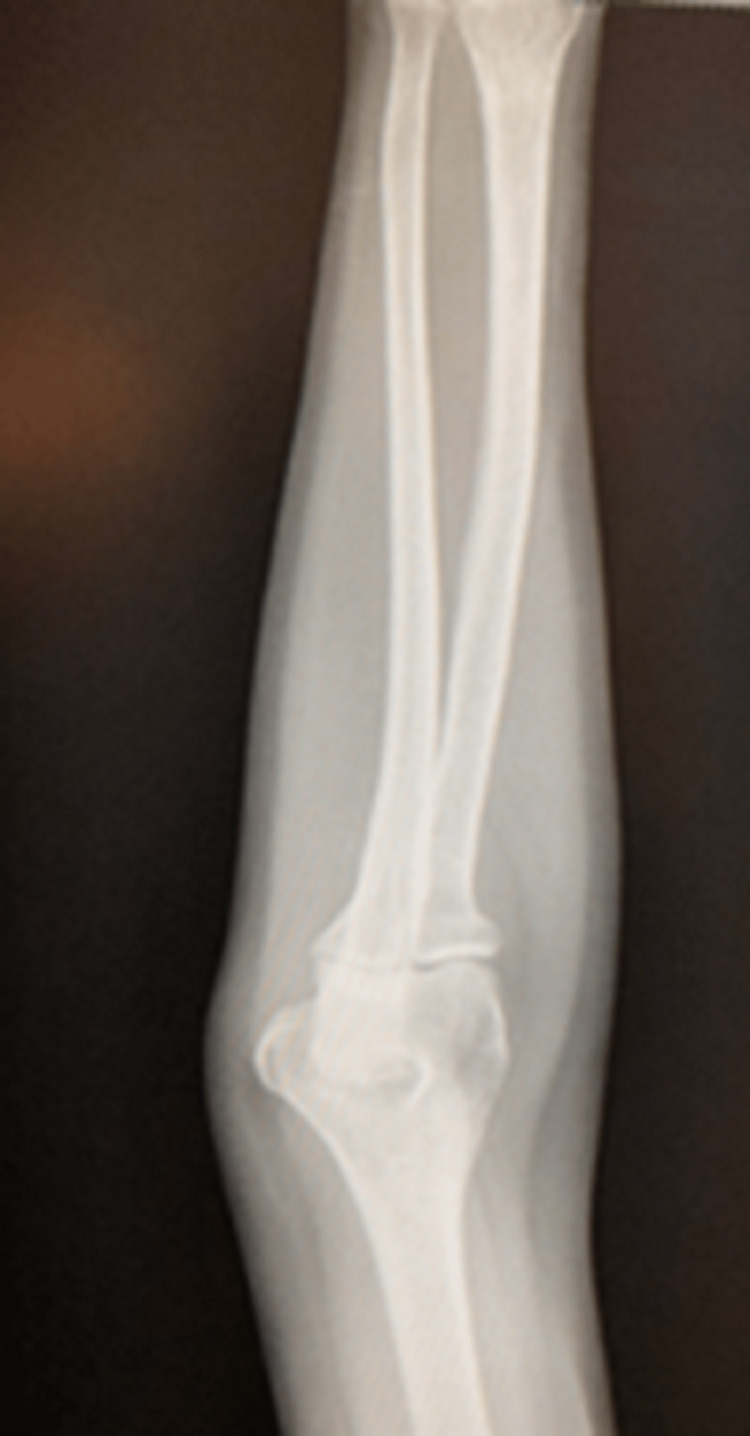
Left elbow radiograph - anterior-posterior view Radiograph of the patient’s left elbow from an anterior-posterior (AP) view. This radiograph does not show any immediate structural defects of the patient’s left radius, ulnar, or distal humerus bones.
